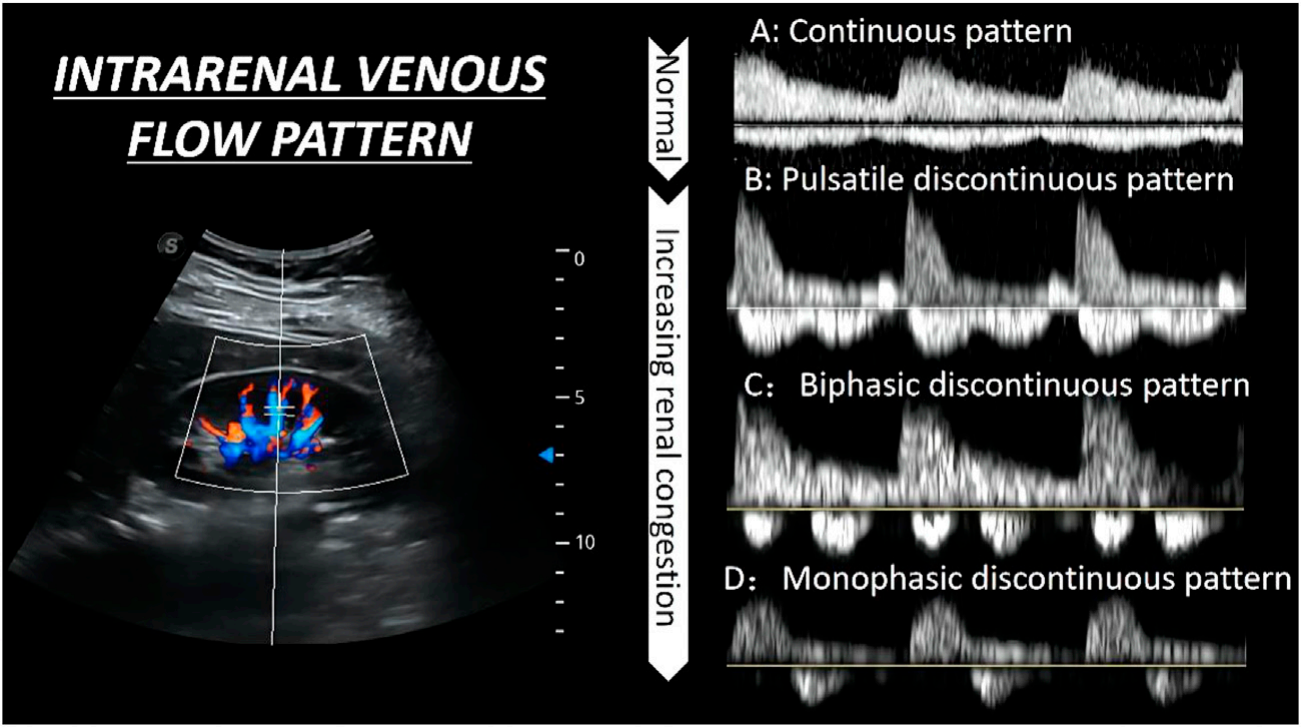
The qualitative evaluation of renal hemodynamics by pulse wave Doppler in the interlobar vessels of the right kidney. The waveform changes are divided into four flow patterns from (A–D).
